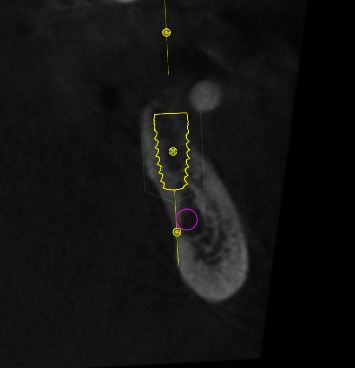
Regio #19-preoperative cross-sectional image from CBCT with visualization of the planned implant position and the inferior alveolar nerve.
